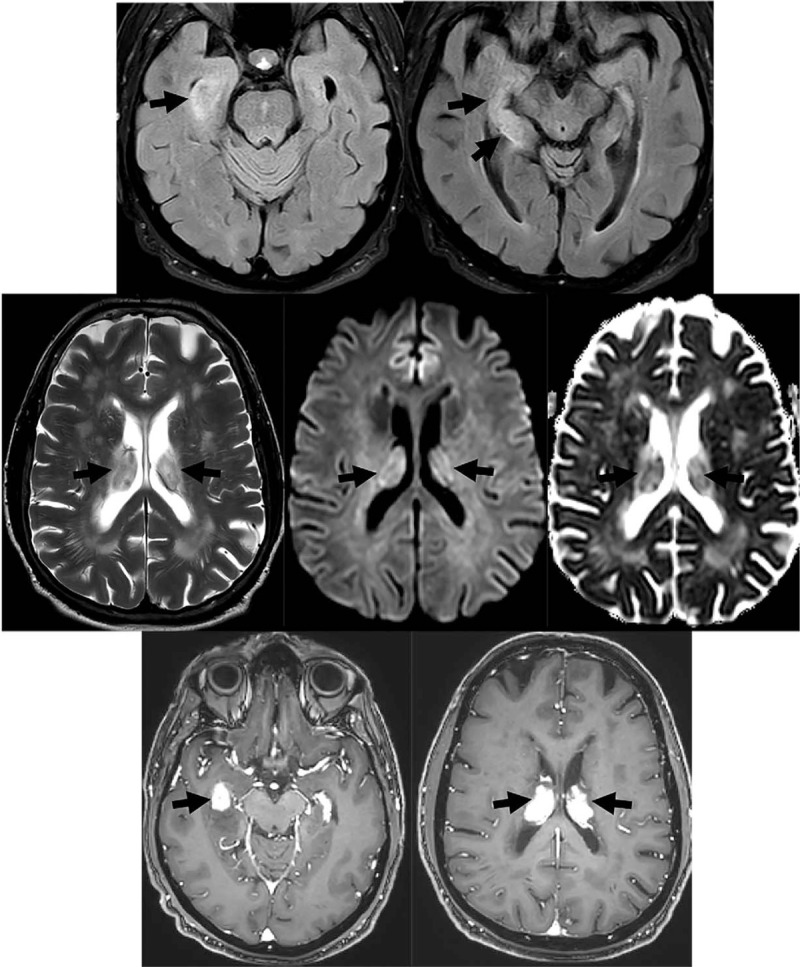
Top row: Axial FLAIR images show increased FLAIR signal in the right medial temporal lobe extending along the right hippocampus, which is mildly enlarged. Middle row: Axial T2, DWI, and ADC map demonstrating additional lesions with mild T2 hypointensity and mild restricted diffusion centered along the inferior aspects of the lateral ventricles bilaterally. Bottom row: Axial post-gadolinium T1-weighted images demonstrating avidly enhancing lesions in the right medial temporal lobe and along the inferior aspects of the lateral ventricles with involvement of the choroid plexus.
